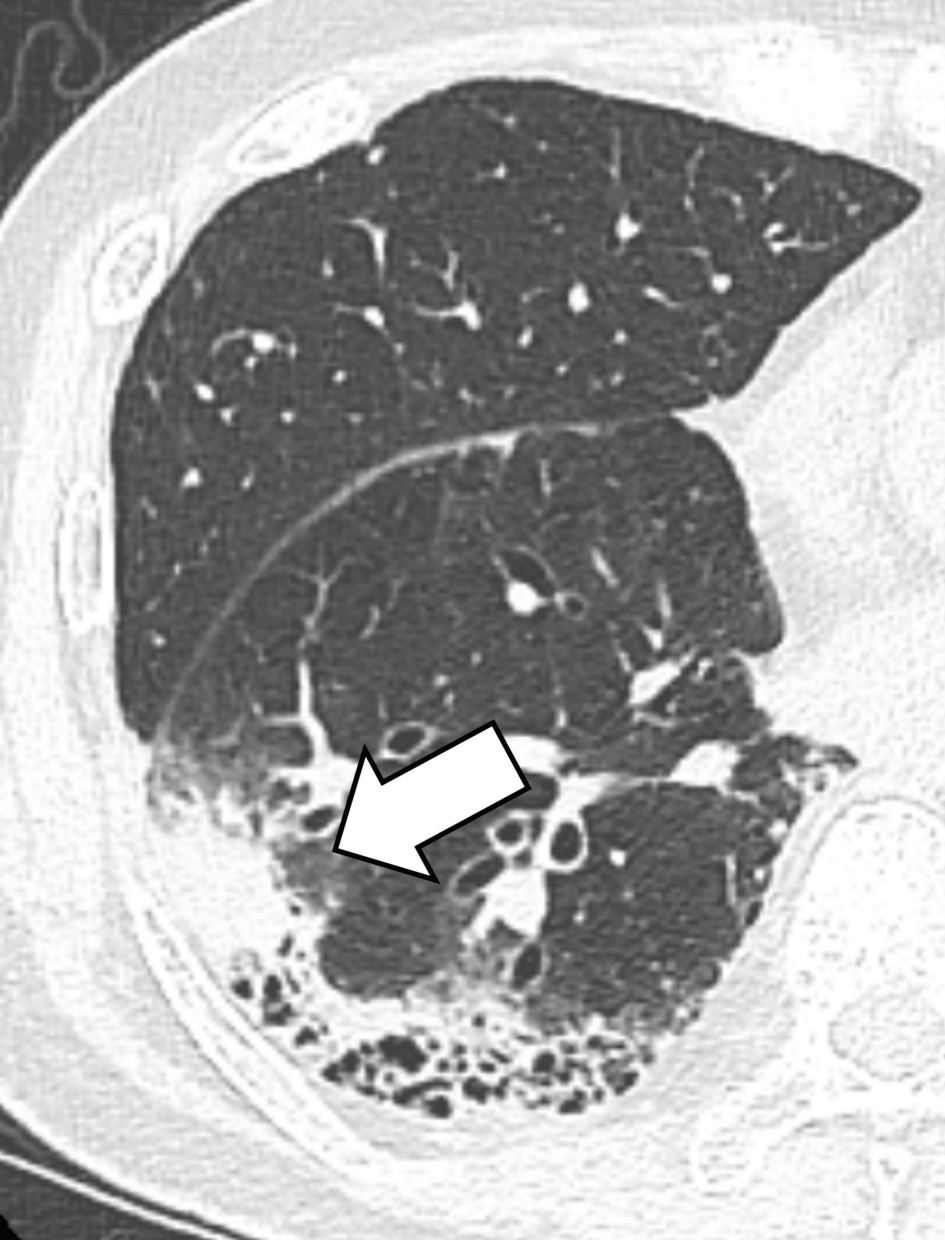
Chest CT before surgery Contrast-enhanced CT scan showing a consolidation with honeycomb cystic changes in the right lower lobe (arrow). CT: computed tomography
